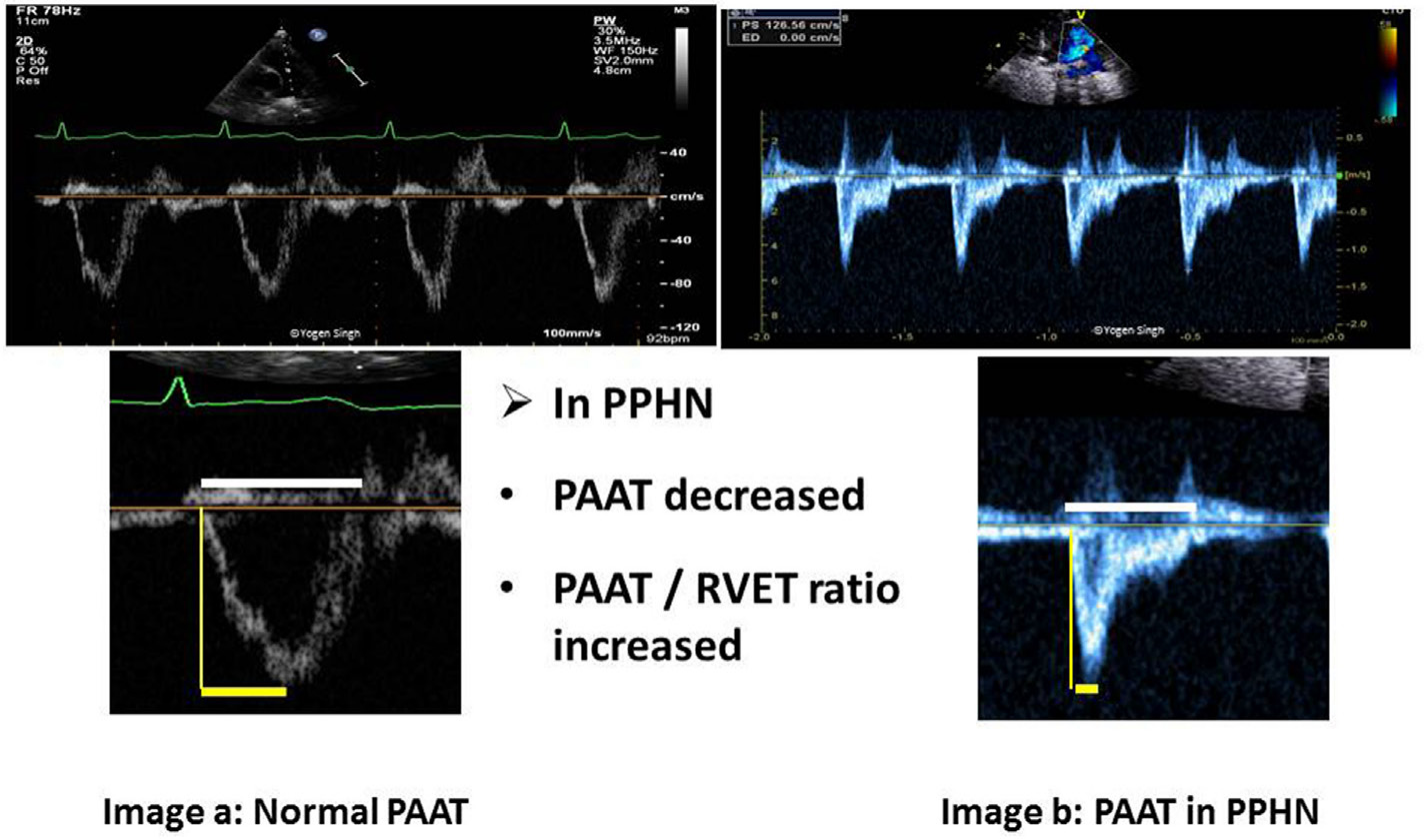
Pulmonary artery acceleration time (PAAT) measurement. Image a shows normal ratio of PAAT and right ventricular ejection time (RVET) in a normal child. Image b shows significantly decreased PAAT and increased PAAT/RVET ratio suggestive of pulmonary hypertension. “Dicrotic notch” may be seen on pulse-wave Doppler spectral in the pulmonary artery.
